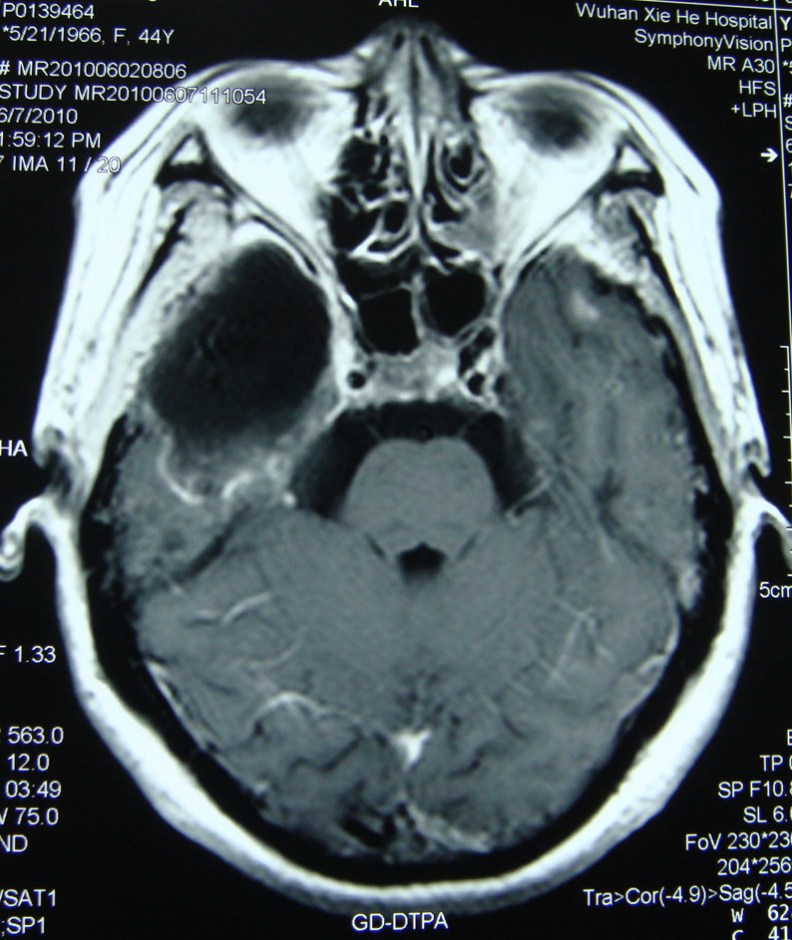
Postoperative axial gadolinium-enhanced T1-weighted images a big cavity in the lower portion of right temporal lobe with surrounding edema, and several minor enhanced lesions in the counterpart of the left temporal lobe.
